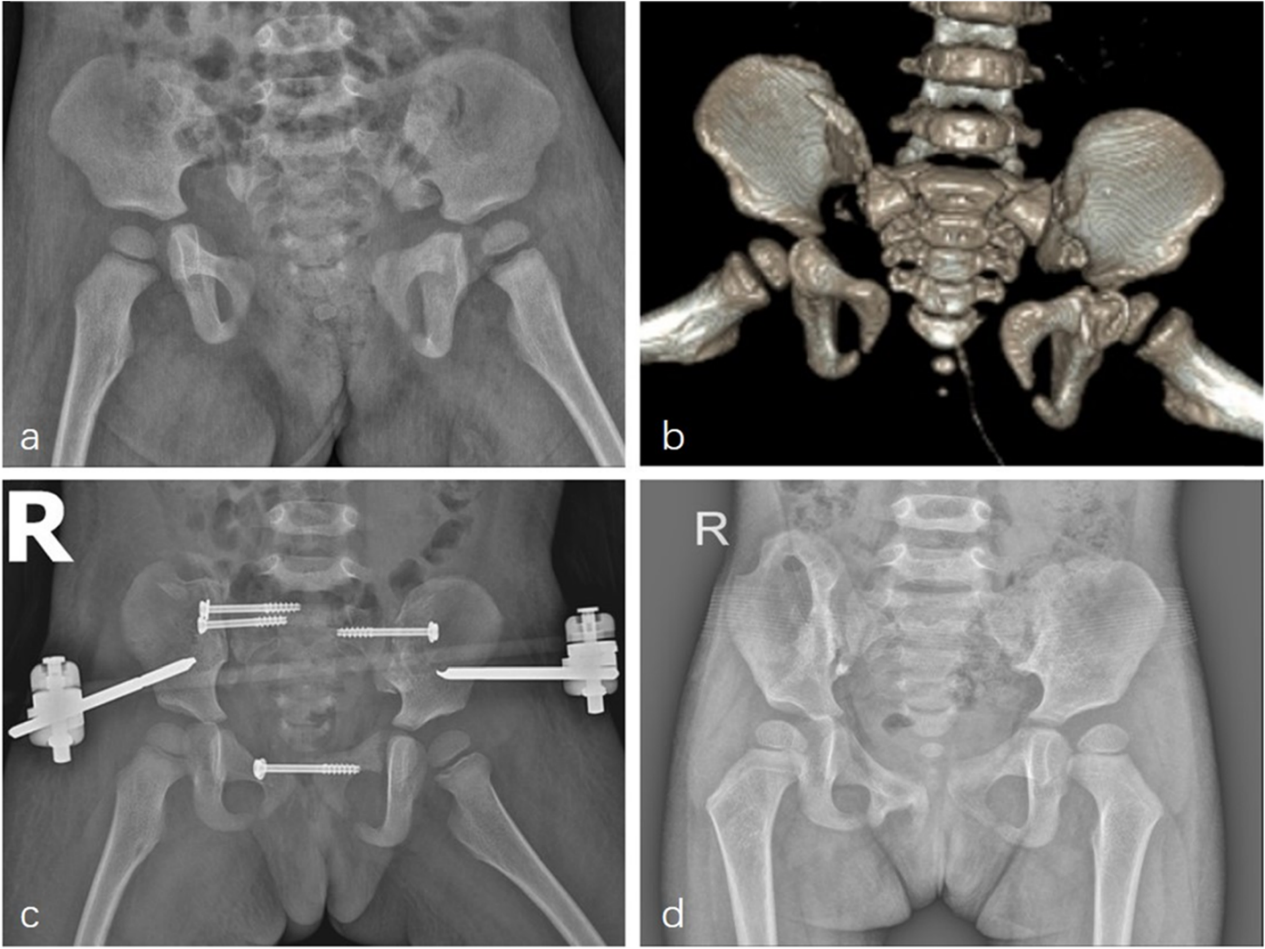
Case 1, a. Preoperative X-ray; b. Preoperative CT with 3 D reconstruction; c. postoperative X-ray; d. X-ray in the final follow-up
